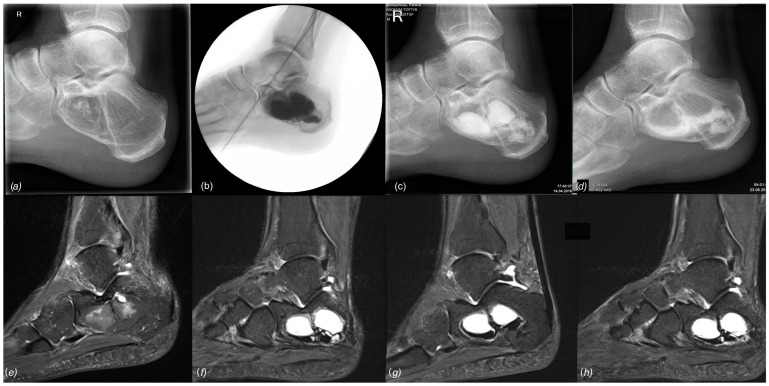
Top, left to right: (a) preoperative X-ray of a large IOL, Milgram Stage 3 (case 22); (b) intraoperative fluoroscopy, postoperative radiographs at 6 (c) and 12 (d) weeks postoperatively showing continued loss of the filling material, consistent with persistent wound drainage. Bottom, left to right: (e) preoperative MRI, (f) sagittal MRI 12 months postop, (g) 20 months postop, and (h) 32 months postop. All MRI are sagittal T1 TIRM weighted.
